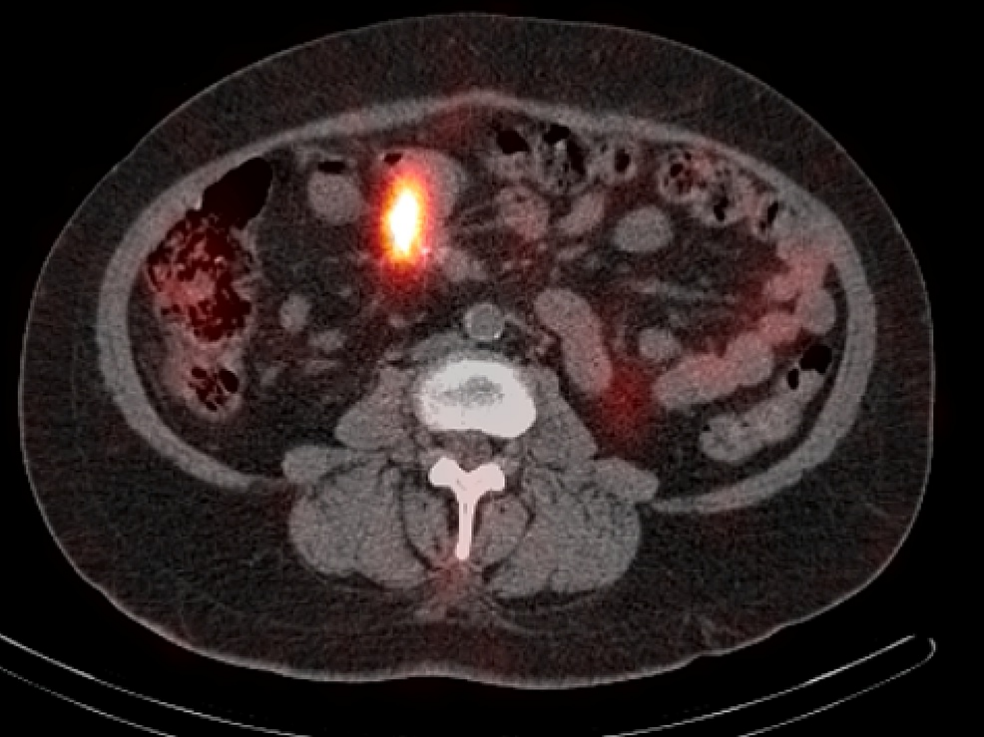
Fused octeriotide uptake scan with SPECT-CT demonstrating a focal radiotracer uptake associated with thickened small bowel lobe and adjacent mesenteric metastasis SPECT, single-photon emission computed tomography.
